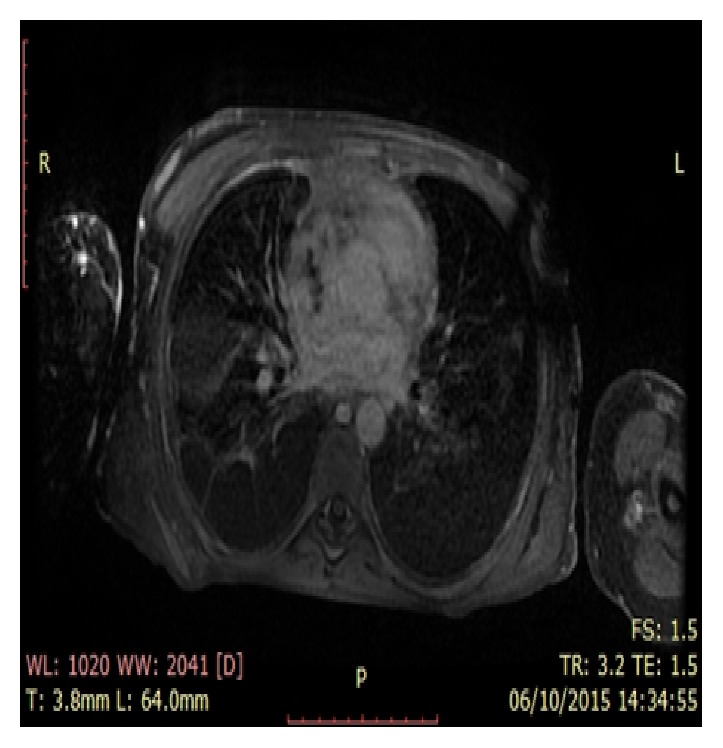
Cardiac MRI, thrombosis superior vena cava. Cross section of the cardiac MRI through the superior vena cava, the white ring shows thrombosis in the superior vena cava.
